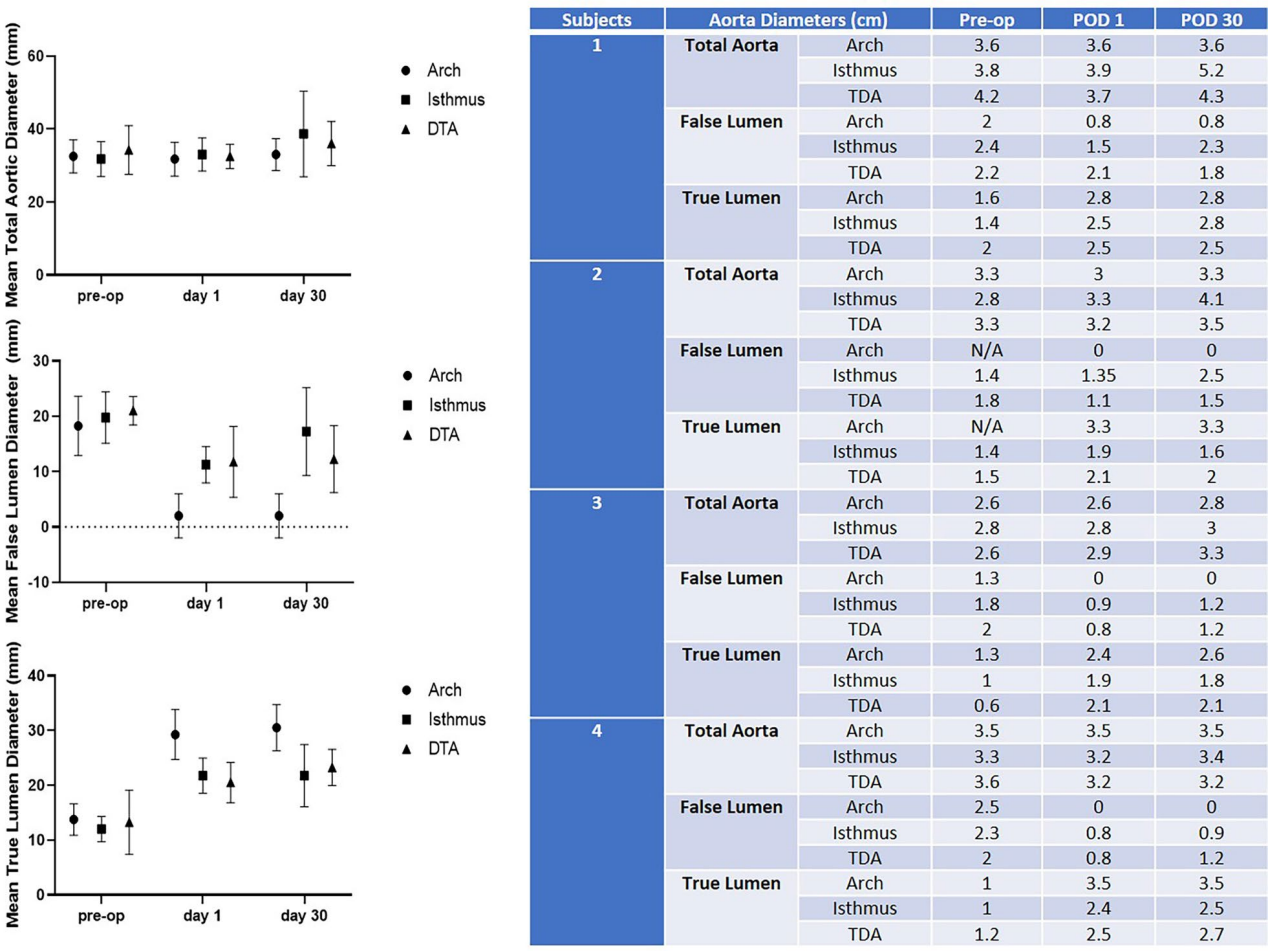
Comparison of the CT measurements of the different aortic diameters
